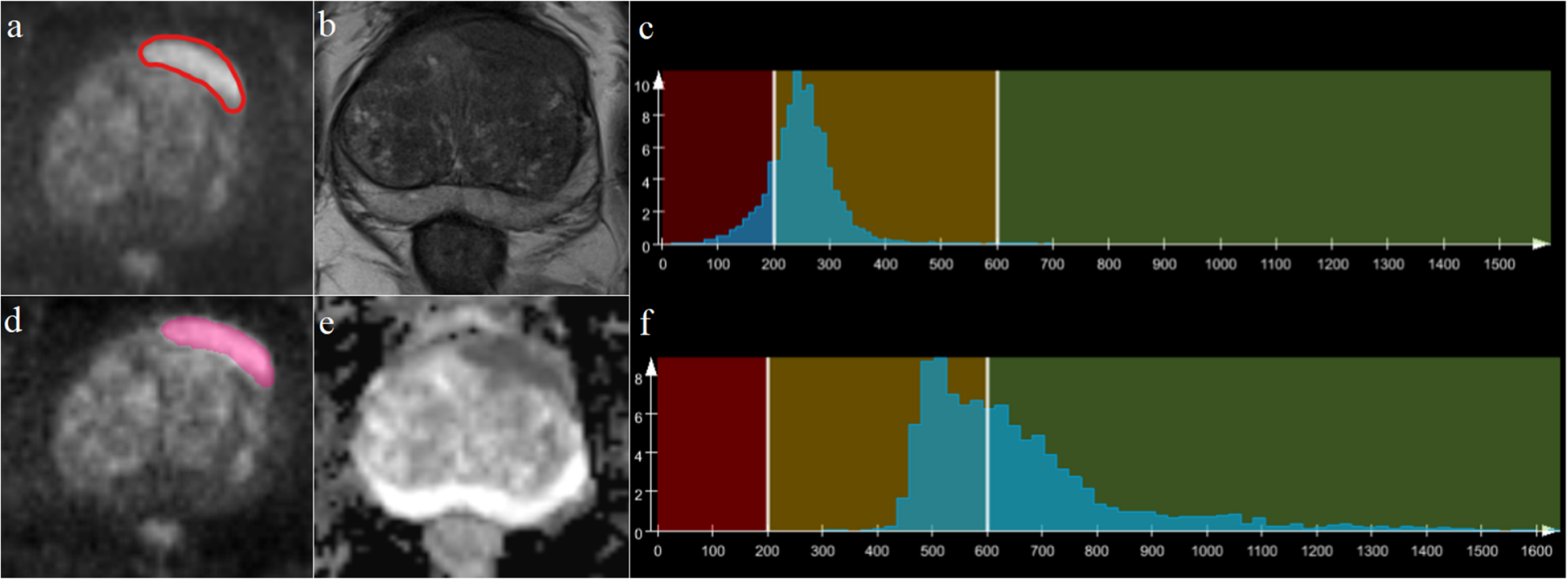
Representative images of a 69-year-old male with prostatic carcinoma (a-f) and Gleason scores of 4 + 3. Segmentation of the lesion is shown on diffusion-weighted imaging (DWI) images with a *b* = 1500 s/mm^2^ (a, d). Histograms (c, f) of T2weighted (T2W) images (b) and ADC maps (e)
